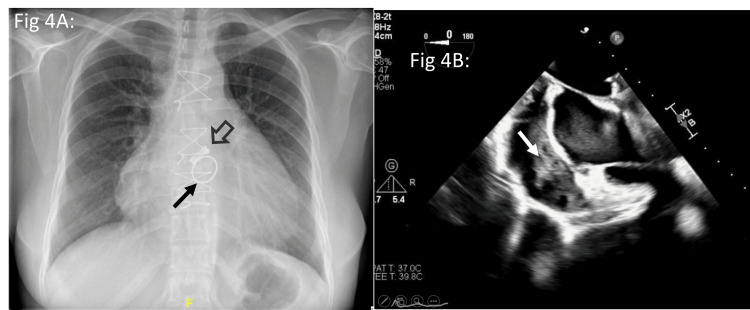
Case 4: Chest X-ray and transoesophageal echo Chest X-ray (4A) showing cardiomegaly, prosthetic aortic (open arrow) and mitral (solid arrow) valves. Transoesophageal echo (4B) showing organised pericardial collection (white arrow).
